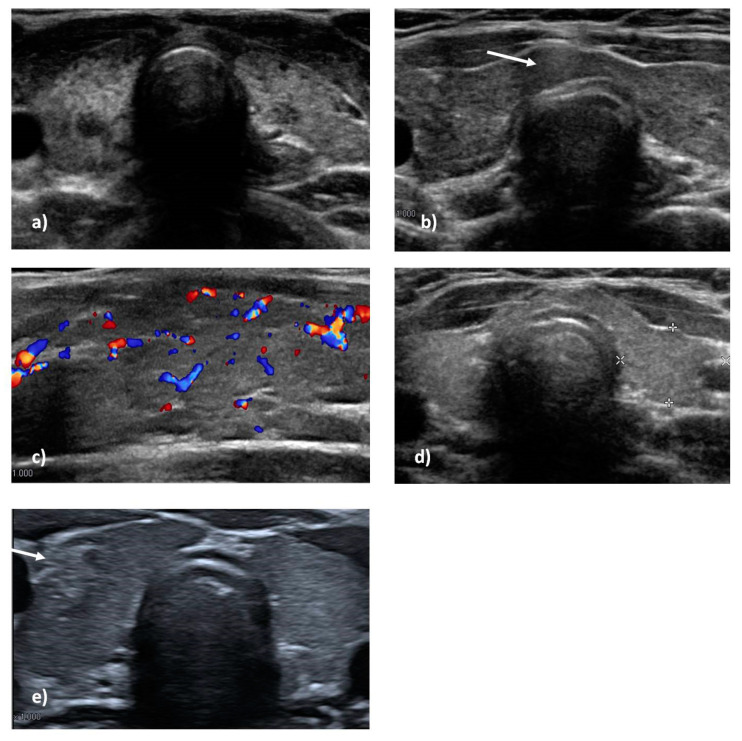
Cervical ultrasonography in some patients with 22q11.2 Deletion Syndrome: thyroid gland hypoplasia as well as inhomogeneity, diffuse hypoechoic areas, and pseudonodular pattern (a); diffuse inhomogeneity, hypoechogenity, and perithyroidal lymph node (white arrow) (b). Increased overflow of thyroid lobe on color doppler ultrasonography (c). Left lobe hypoplasia (d); right thyroid lobe neoplasia in a patient with Graves’ disease (white arrow) (e).
